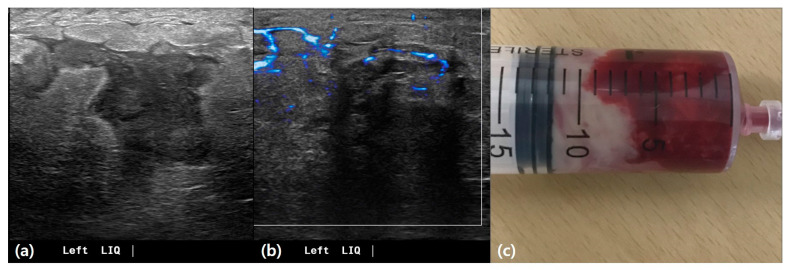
Idiopathic granulomatous mastitis with abscess. A 35-year-old female patient presented with breast discomfort persisting for 14 days. (a) Ultrasound imaging demonstrates a hypoechoic lesion with diffuse skin thickening and edematous changes in the lower inner quadrant of the left breast. (b) Doppler ultrasound imaging reveals a moderate increase in blood flow, shown in blue. (c) Ultrasound-guided needle aspiration was performed, and 12 cc of bloody pus was aspirated.
